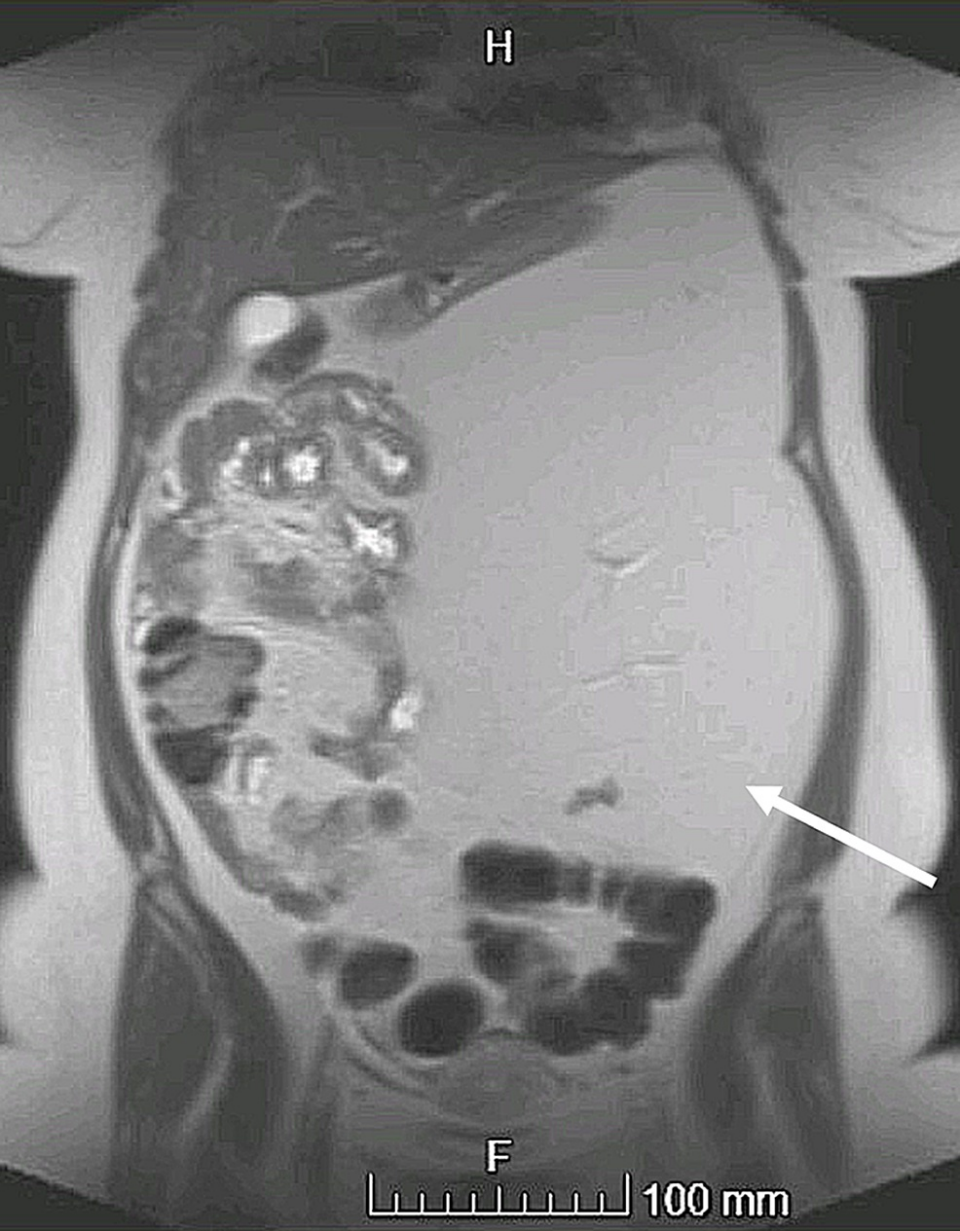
MRI abdomen and pelvis with contrast showing a large abdominal mass (arrow) in the left side of the abdominal cavity with homogeneously high in T1 and T2 WI and homogenous drop of the signal in the fat-sat sequences.
